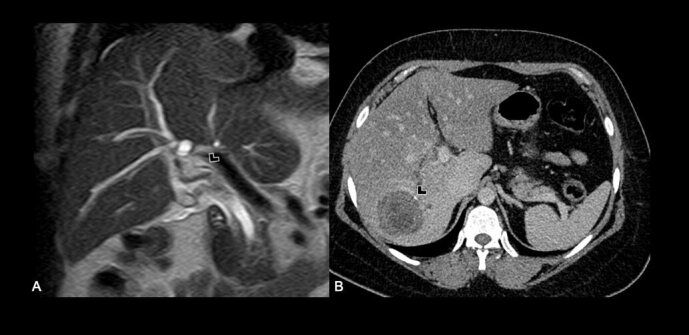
(A) Intrahepatic and extrahepatic bile duct dilatation with endoluminal hydatid sand; Coronal T2-weighted image; (B) Complex cystic lesion with isolated peripheral calcifications; Axial contrast – enhanced computed tomography.
